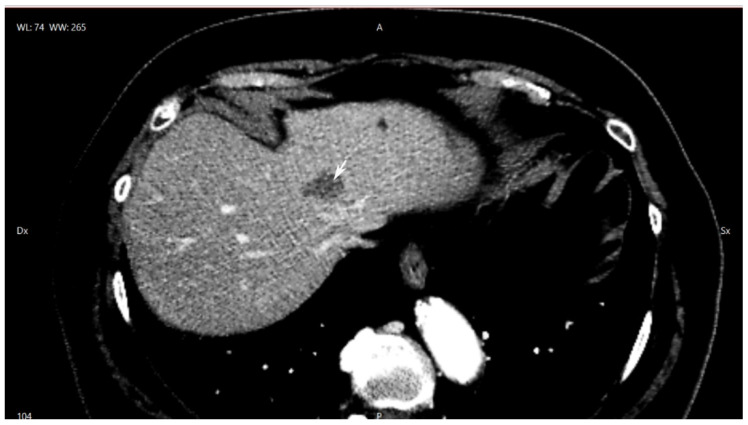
Upper portion of liver CT scan: lesion at the origin of the common hepatic trunk. (white arrow: lesion).
